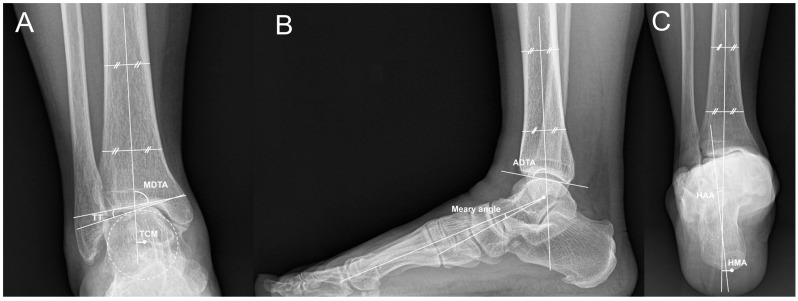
Weight-bearing ankle anteroposterior radiograph showing measurements of the medial distal tibial angle (MDTA), the talar tilt (TT), and the talus center migration (TCM) (A). Weight-bearing foot lateral radiograph showing measurements of the anterior distal tibial angle (ADTA) and the Meary angle (B). Hindfoot alignment radiograph showing measurements of the hindfoot alignment angle (HAA) and the hindfoot moment arm (HMA) (C).
